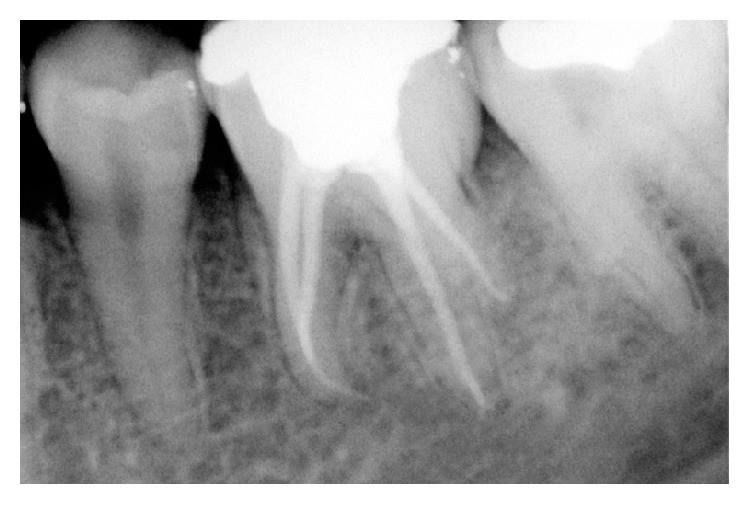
Postoperative radiograph of obturation.
